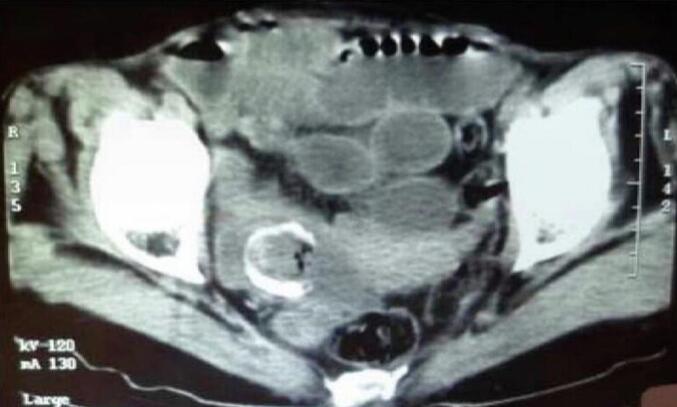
CT scan showing impacted gallstone in ileum (second case).
